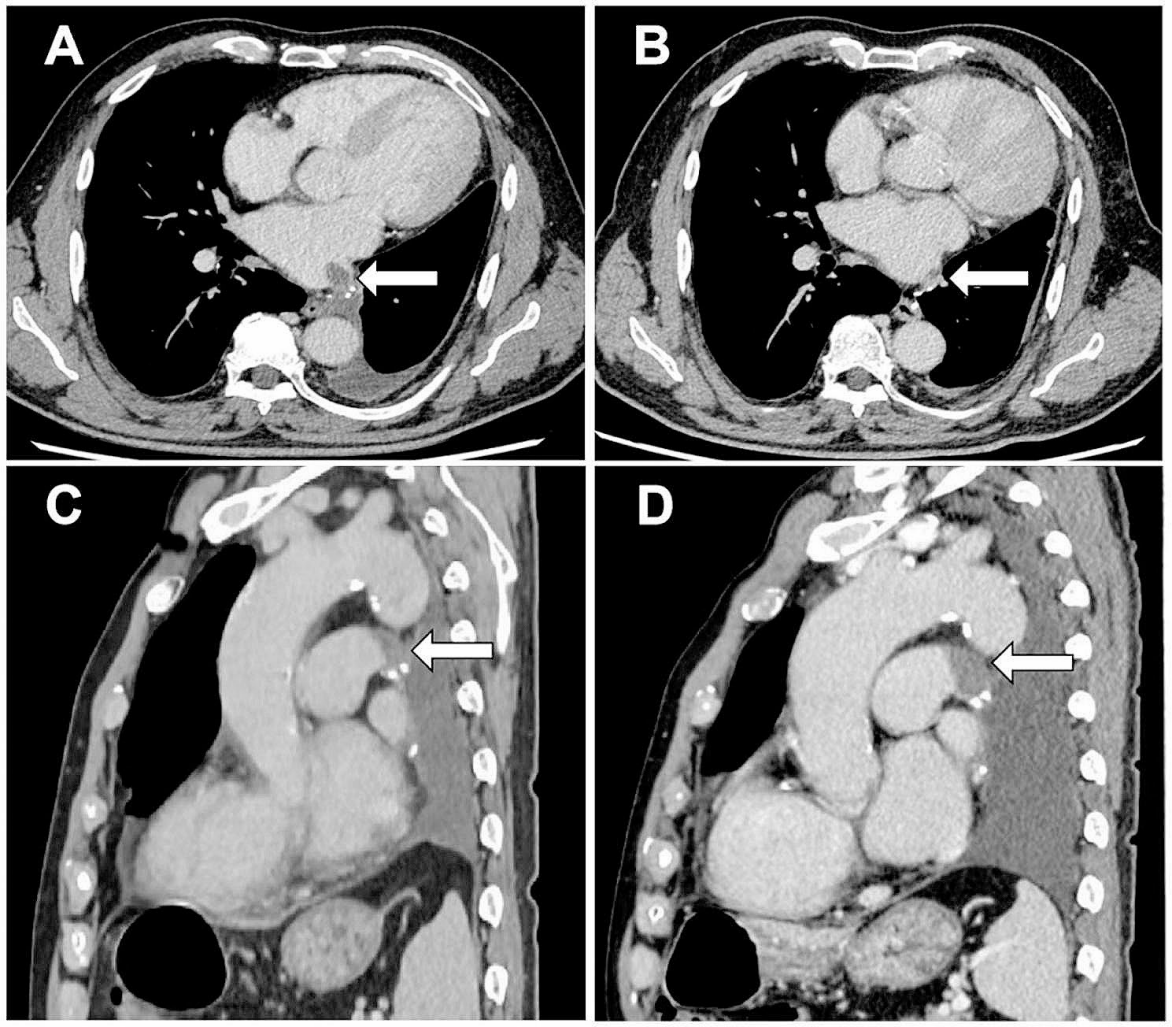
Evolution of pulmonary vascular stump filling defects over follow-up. (A) Initial detection of a convex-shaped filling defect in the left inferior pulmonary venous stump. (B) Subsequent follow-up CT scan 232 days later revealing partial spontaneous resolution of the filling defect (white arrow indicates the vascular stump). (C) Identification of a concave-shaped filling defect in the left main pulmonary arterial stump. (D) Progressive increase in the filling defect observed 169 days post-onset (white arrow indicates the vascular stump)
